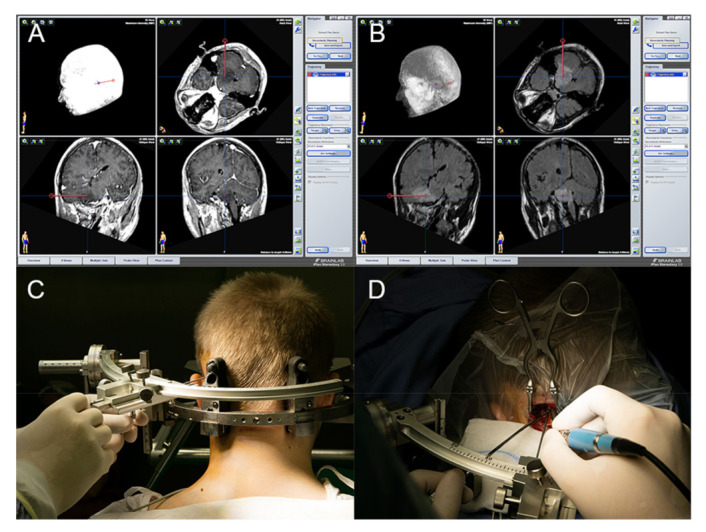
(A) T1-weighted image of a tumor located in the pons, cerebellar peduncle, and cerebellar hemisphere. (B) Fluid attenuated inversion recovery magnetic resonance imaging (FLAIR MRI) of a tumor located in the pons, cerebellar peduncle, and cerebellar hemisphere. (C) Patient with a stereotactic frame. (D) Suboccipital burr holes made using a high-speed drill.
